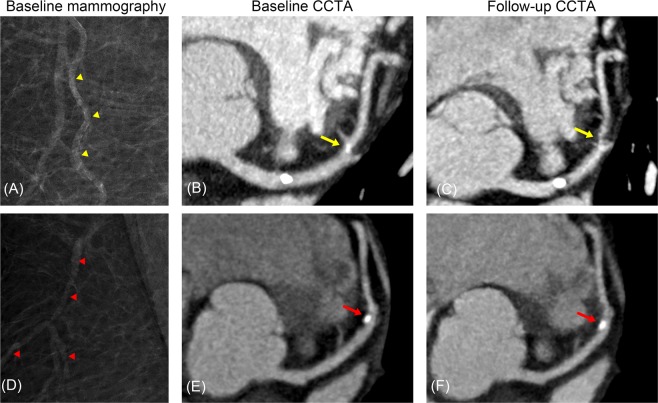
Representative cases. A 63-year-old asymptomatic woman with a BAC score of 5 (yellow arrow heads) on screening mammography (A) demonstrated calcified plaque without significant stenosis (percent diameter stenosis, 10–20%) at the proximal LAD and mixed plaque (percent diameter stenosis, 10–20%) at the mid LAD (yellow arrow). (B) Three years later, she was referred to the emergency department with chest pain and underwent CCTA, which demonstrated progression of the mid LAD lesion (percent diameter stenosis, 90%; yellow arrow). (C) Invasive angiography also demonstrated the tight stenosis of the mid LAD (percent diameter stenosis, 90%) and percutaneous coronary intervention was performed. A 51-year-old asymptomatic woman without evidence of BAC (red arrow heads) on screening mammography (D) demonstrated calcified plaque (percent diameter stenosis, 30%) at the mid LAD (red arrow). (E) Five years later, she visited the outpatient clinic due to epigastric pain and underwent CCTA, which demonstrated no change in the mid LAD lesion (red arrow). (F) BAC, breast arterial calcification; CCTA, coronary computed tomographic angiography; LAD, left anterior descending artery.
